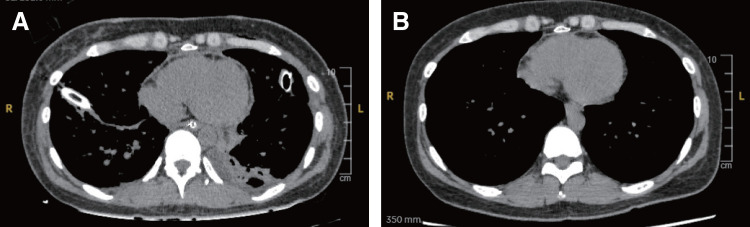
(A) CT scan during acute lung rejection after transplantation. (B) CT scan at 6 months postoperatively. CT: computed tomography
